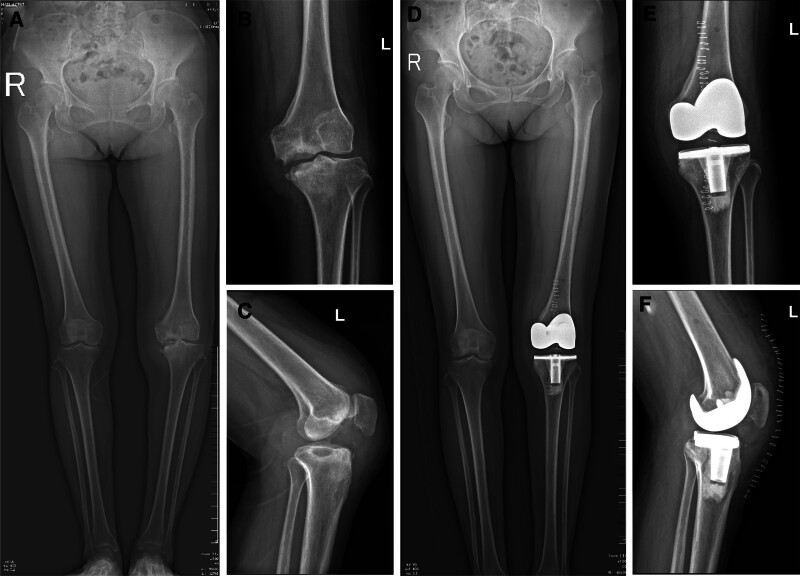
(A–F) Radiographic progression of the left knee: (A) Preoperative full-length bilateral lower extremity radiograph. (B) Preoperative anteroposterior projection radiograph demonstrating severe osteoarthritis, there were different degrees of bone cumbersomening at the edges of the articular surfaces with joint space narrowing. (C) Preoperative lateral projection radiograph. (D) Postoperative full-length bilateral lower extremity radiograph. (E) Postoperative anteroposterior projection radiograph demonstrating the artificial prosthesis was in a good position without loosening or fracture. (F) Postoperative lateral projection radiograph.
